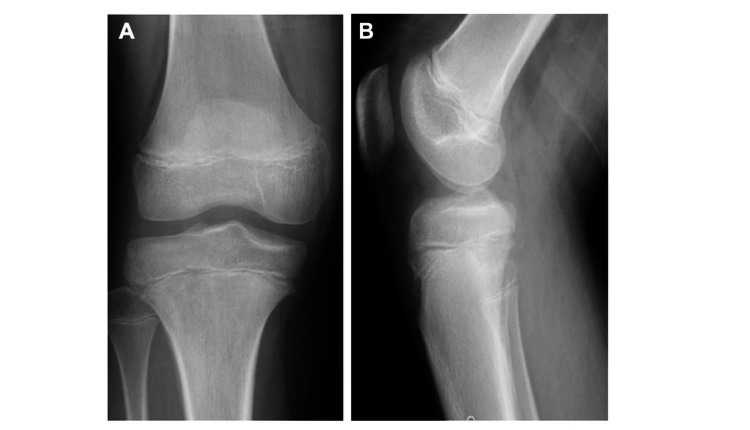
X-ray of the right knee: (A) Anteroposterior view and (B) lateral view. No significant bone destruction or sclerosis is observed.
